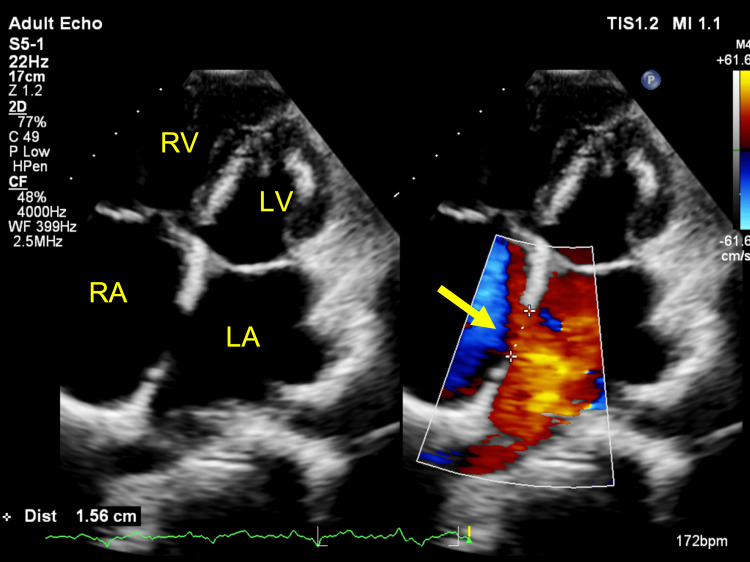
Two-dimensional TTE, apical 4-chamber view with color flow Doppler, systolic phase, demonstrates a large ASD (arrow) with a longitudinal diameter of 15.6 mm. TTE: Transthoracic echocardiography; ASD: Atrial septal defect.
